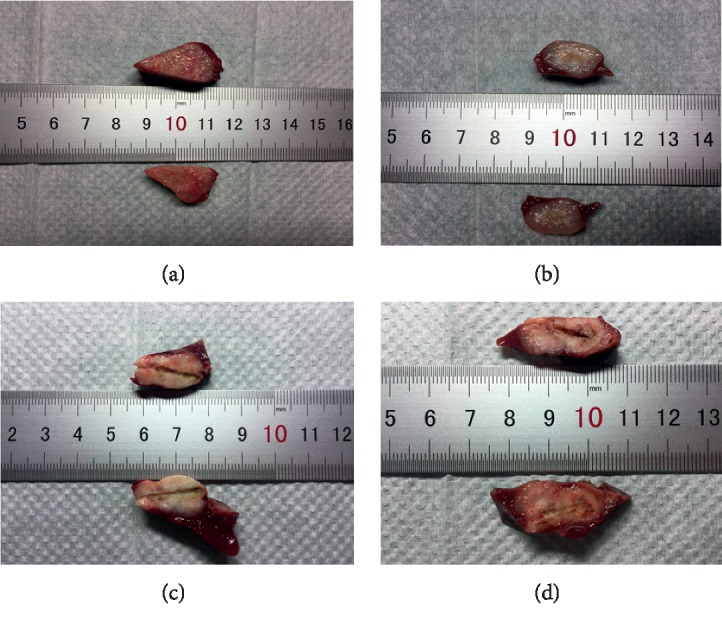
Macroscopic view of liver tumors after treatments. (a) The control group; (b) microbubble-enhanced ultrasound alone; (c) MWA ablation alone; (d) MEUS + MWA ablation. Tumor margins and necrotic margins are difficult to distinguish.
